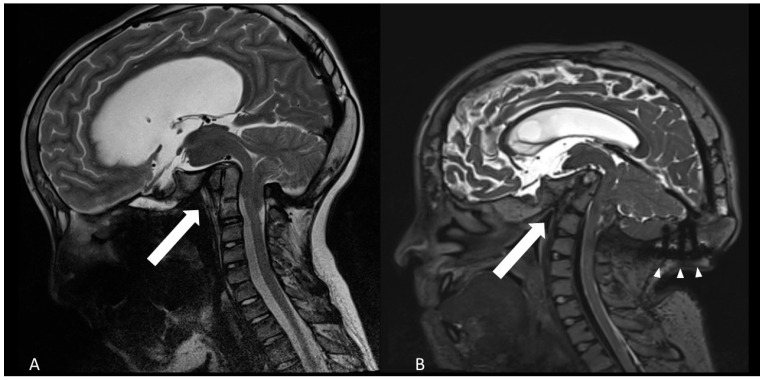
Progressive basilar invagination and flattening of the calvaria as shown by sagittal T2-weighted MRI. (A): age of 12 years (B): 18 years old. The arrows point to the angle between clivus and dens axis, diminishing from about 75° to 50°. Arrowheads show the occipital instrumentation.
